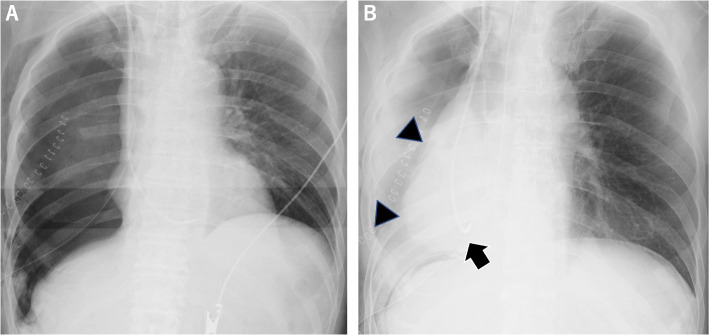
Postoperative chest radiograph. A A routine chest radiograph taken in the operating room has unremarkable findings. B A chest radiograph taken postoperative day one reveals a shift of the heart to the right thoracic cavity (arrowhead) with an abnormal positioning of the Swan-Ganz catheter (arrow)
